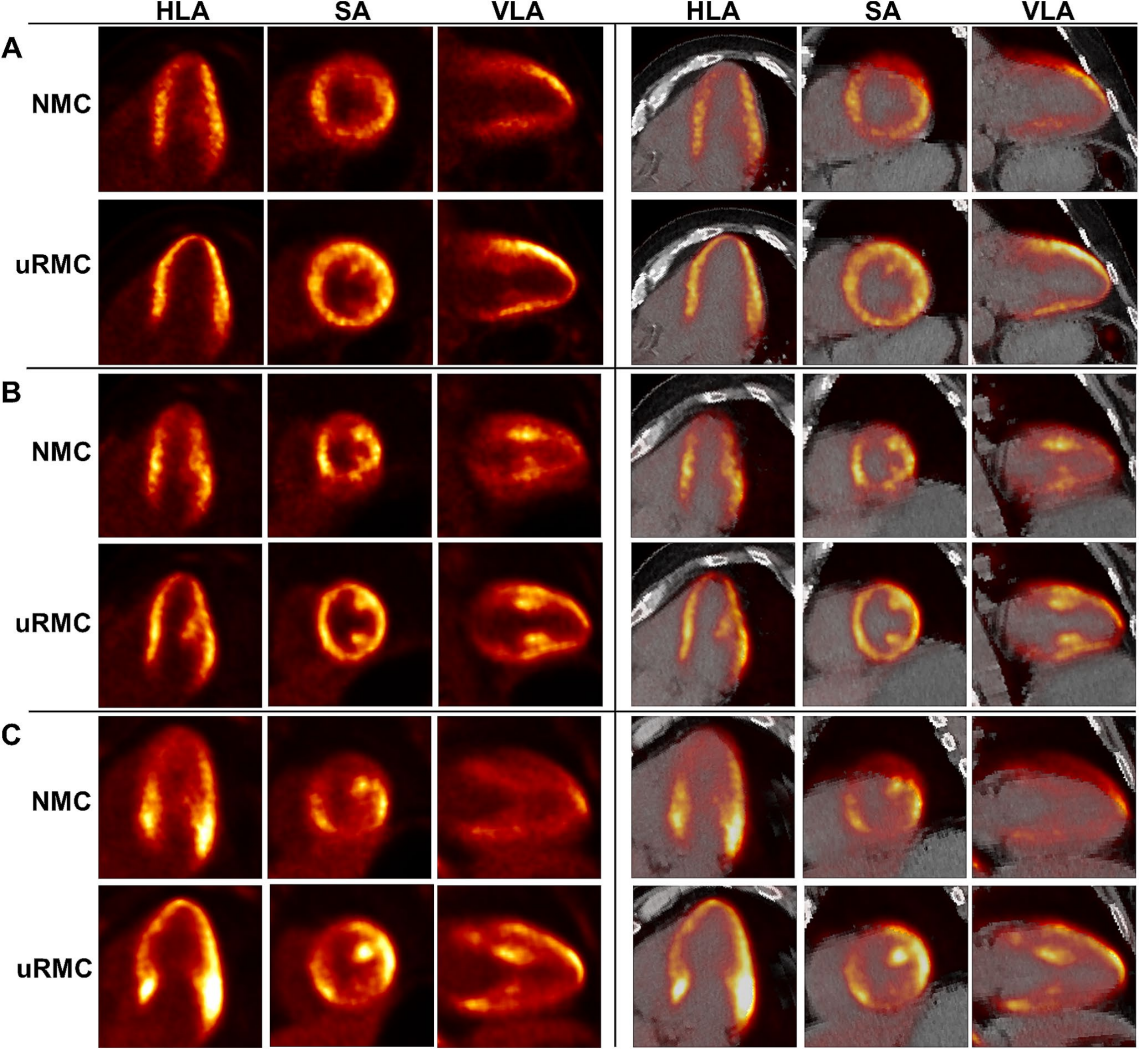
Cardiac PET and PET/CT fusion images from three cases comparing NMC and uRMC images. uRMC shows sharp delineation and PET-CT alignment of ventricular wall and interventricular septal [^18^F]FDG accumulations by eliminating respiratory-motion-induced blurring. NMC: no motion correction; uRMC: unified respiratory motion correction
